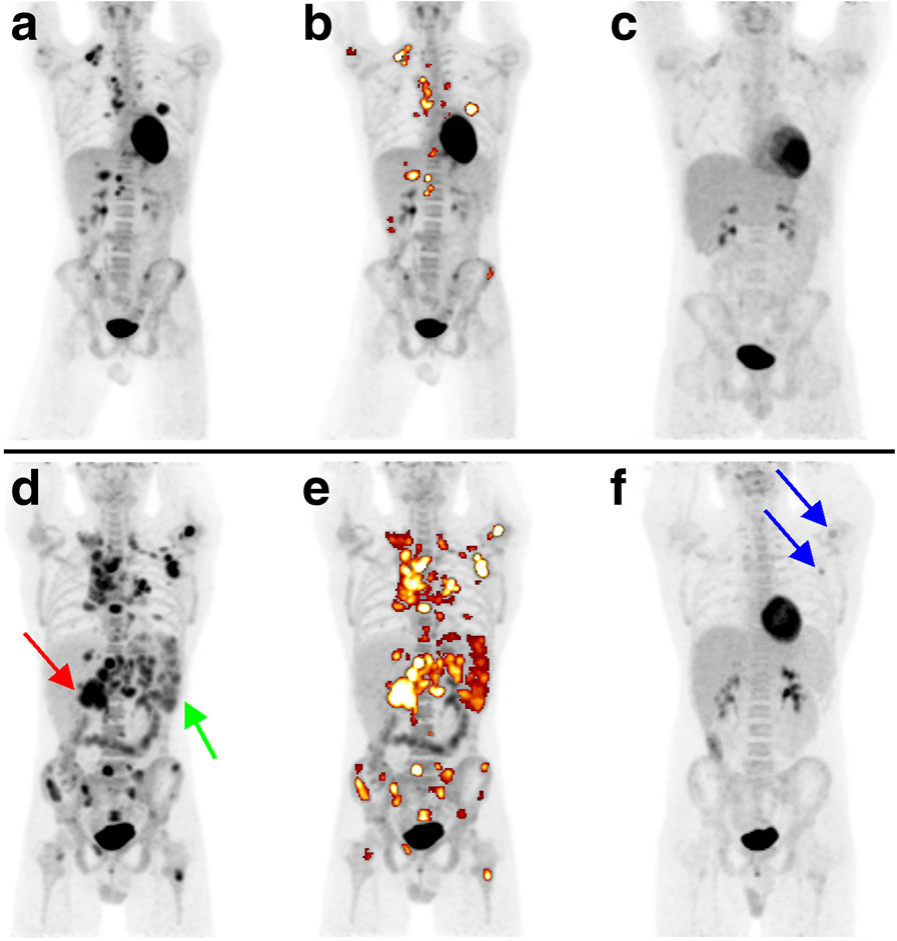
Patient examples of low and high MTV. Representative examples of FDG-PET maximum intensity projections (MIP) of two patients with stage IV disease before induction therapy (a, b + d, e) and at ERA (c, f). In the middle column (b, e), the delineated pretherapeutic MTV is colored (high activity: white, low activity: brownish). a-c: A 17-year-old male with stage IV disease (liver, lung) and AR who had a low MTV (51 ml). d-f: A 17-year-old male with stage IV disease (skeletal) and IR who showed a high MTV (792 ml); please also note the large lymph node mass at the liver hilus (*red arrow*) and extensive splenic involvement (*green arrow*). At ERA, considerable FDG uptake (Deauville score 4) can still be detected especially in a left axillary lymph node and the left humerus (*blue arrows*)
